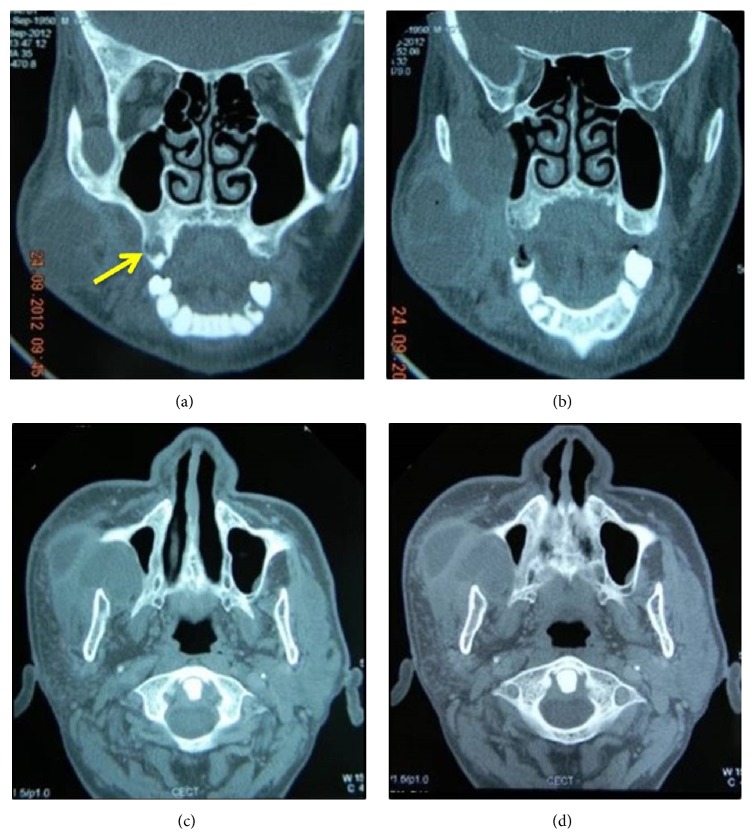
((a) and (b)) Posteroanterior view; ((c) and (d)) transverse CT at C 6 level: CT scan showing a well-defined loculated soft tissue mass in the buccal space. The lesion shows multilocularity with gel or fluidic content. The lesion is seen compressing the maxillary bone in the area of antral wall laterally.
